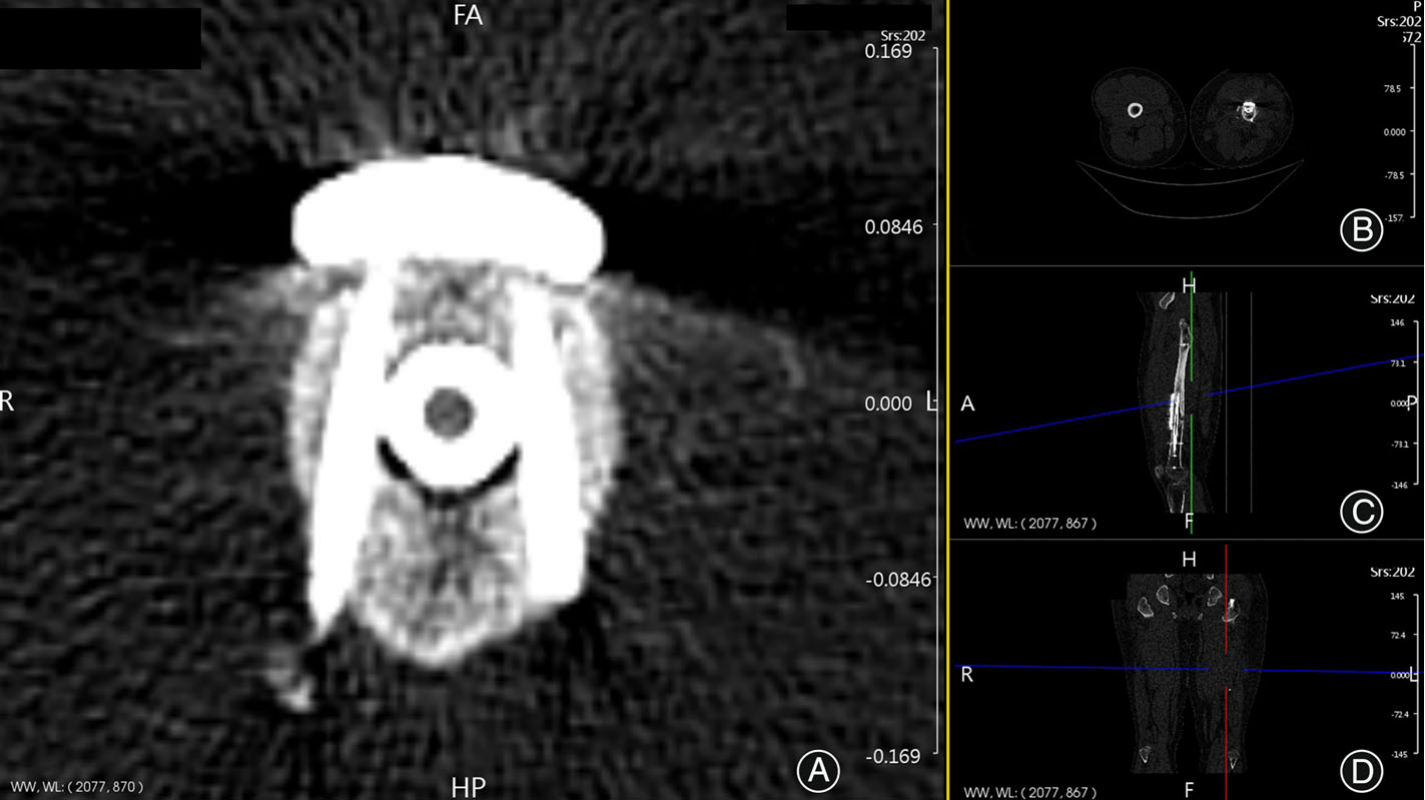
Three‐dimensional CT reconstruction of multidimensional cross locking plate (MDC‐LP) bi‐cortical screw fixation with original intramedullary nail (IMN) during postoperative follow‐up. (A) Coronal section of MDC‐LP with bi‐cortical screw fixation clamping the original IMN on femoral shaft during 3D reconstruction. (B) Transverse scanning image during 3D reconstruction. (C) Sagittal section image during 3D reconstruction. (D) Coronal section image during 3D reconstruction
